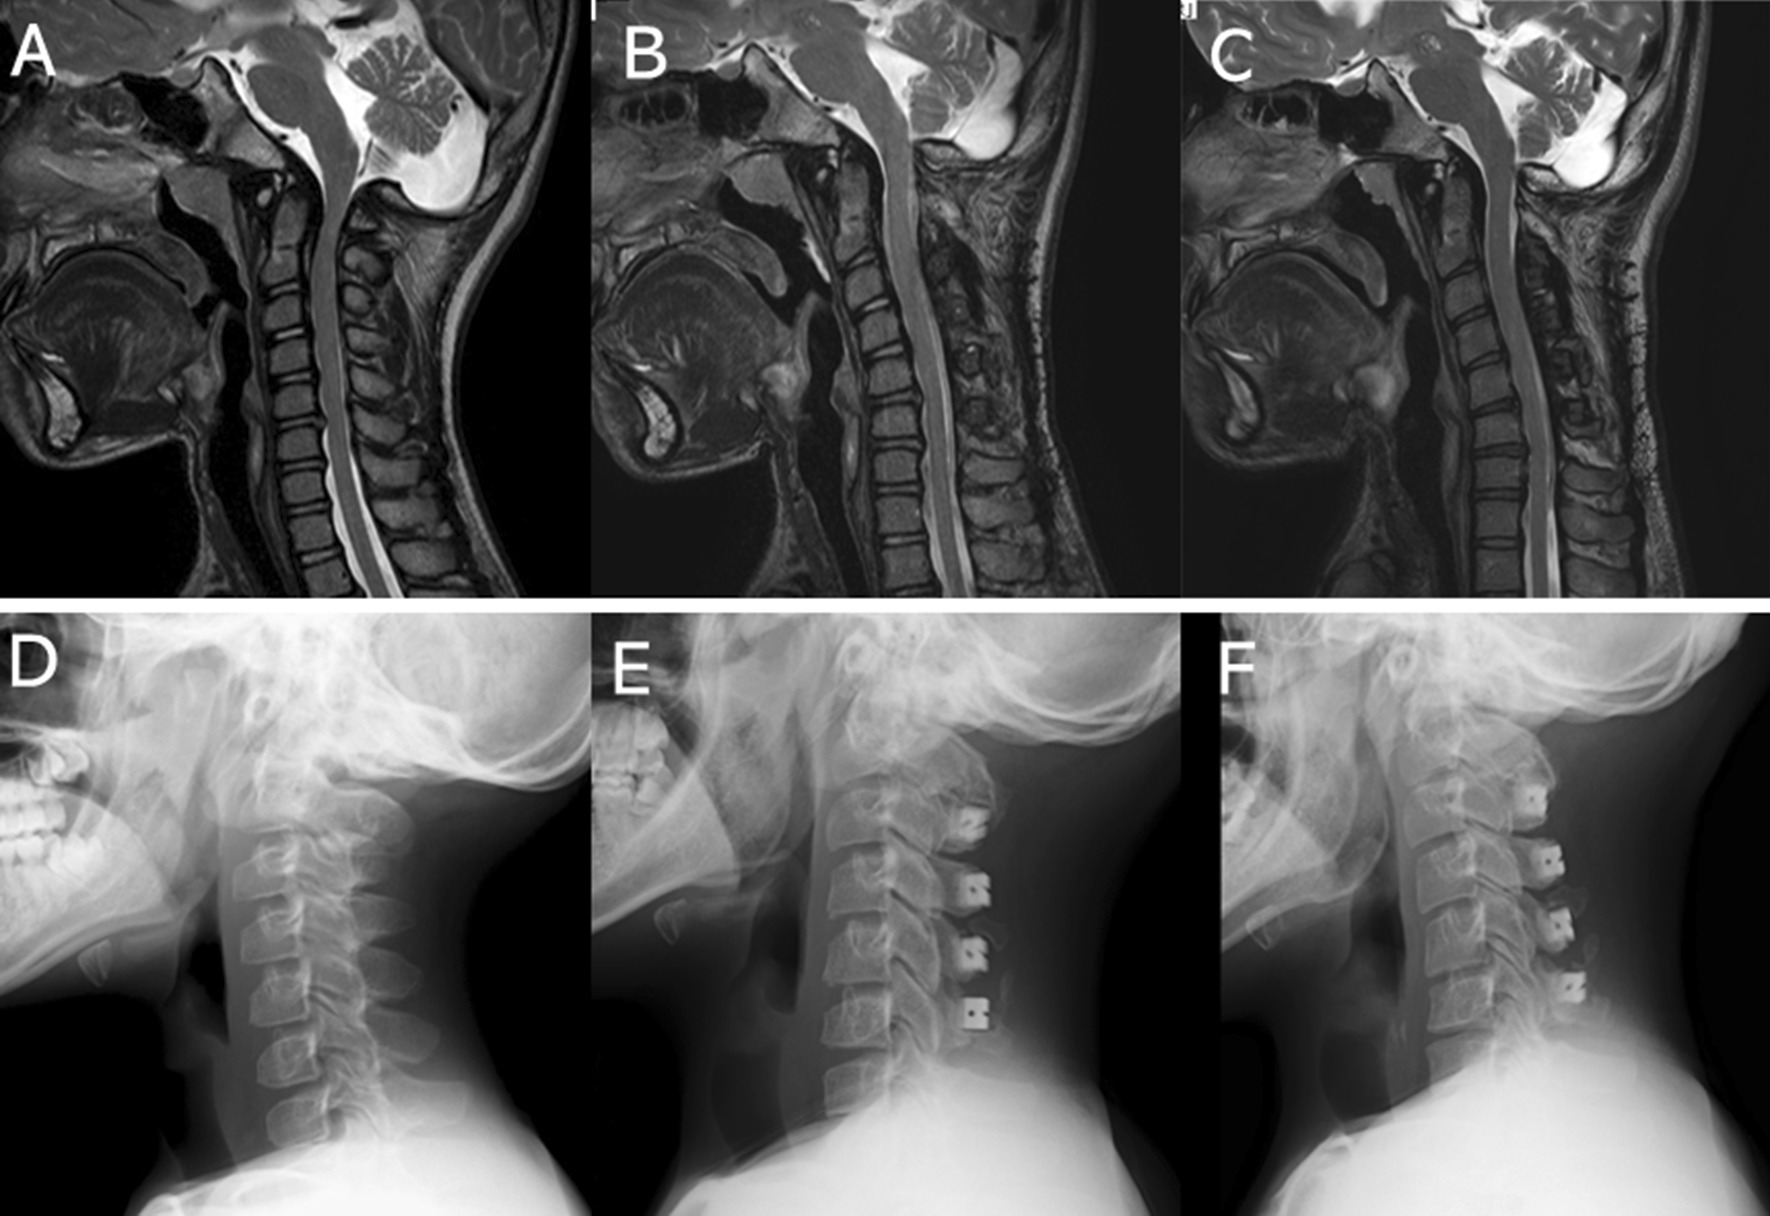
T2WI sagittal images and plain lateral radiographs of a 13-year-old male patient with MPS type II. The patient experienced bilateral hand numbness when his neck was extended. A, D Before operation. B, E Two years postoperatively. C, F Eight years postoperatively. Symptom disappeared postoperatively. Note that the cervical kyphosis gradually progresses in 8 years without clinical symptom exacerbation. *MPS* mucopolysaccharidoses
